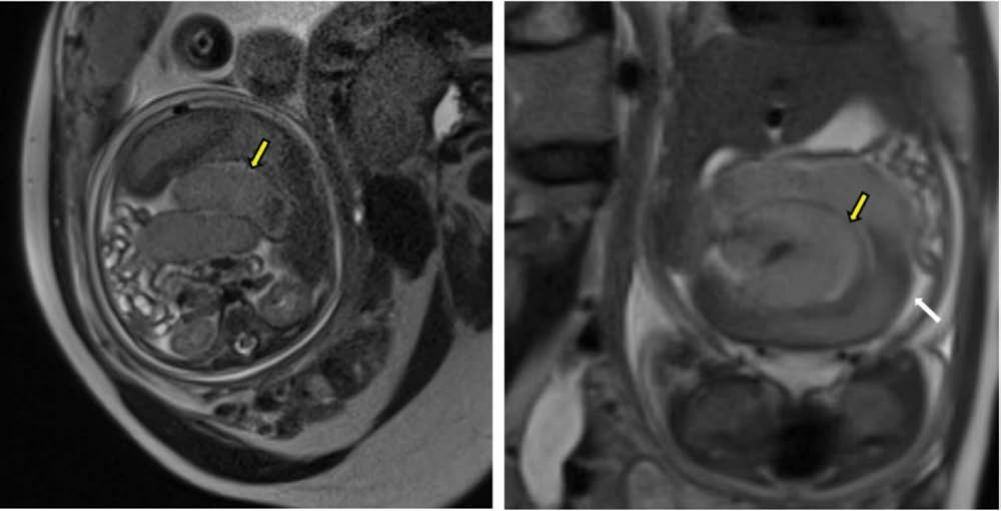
The fetal MRI Showed that the intestinal was spirally dilated (yellow arrow), and the ascites was visible (white arrow). MRI = magnetic resonance imaging.
